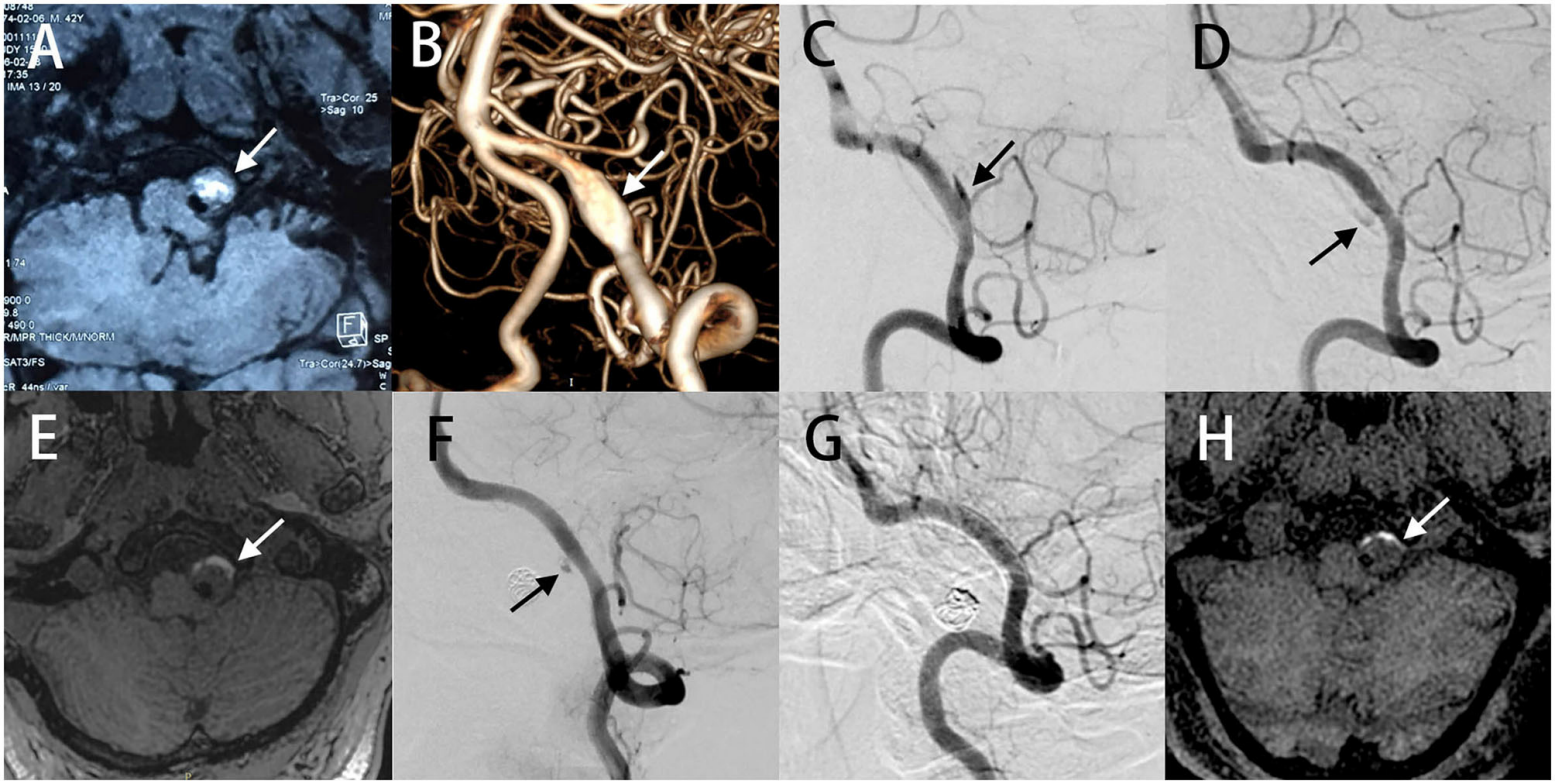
MR image detected a partially thrombosed aneurysm adjacent to the left part of medulla (A). 3D DSA image demonstrated a VADA in the fourth segment of the left VA (B). Angiography at 5 months follow-up revealed that the stent were patent with partial aneurysm residues (C). Partial residual aneurysm of the left VADA was observed on 14 months (D) and 2 years (F) follow-up angiography, and complete occlusion on 3 years (G). The volume of the aneurysm was not significantly changed from 14 months (E) and 2 years follow-up MR images (H).
